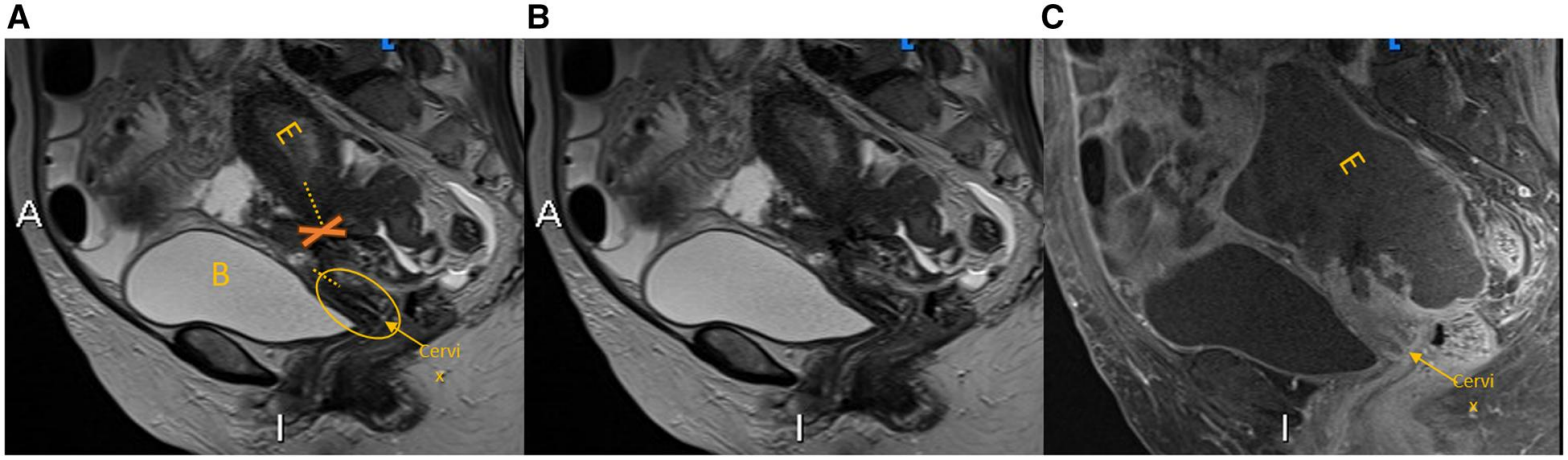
Day 12 of admission MRI pelvis with contrast—sagittal slices. (A and B) *T2-weighted sagittal sequence, both annotated and unannotated*. A (annotated) demonstrates the high T2 signal bladder anteriorly, with the Uterus and T2 bright Endometrial cavity (E) posterior and superior to the bladder. The “X sign” is again appreciable with uterine and adnexal structures tethering between the misaligned uterus and cervix. On A, the cervix and uterine body/fundus (E), do not appear to normally align, with discontinuation of the alignment represented by the dotted lines. (C) *Post-contrast sagittal T1-weighted fat suppressed image at the slice as A and B*: The cervix has again been labelled. The key finding on this image, is the total lack of normally expected uterine and uterine mass enhancement, whereas the misaligned cervix continues to demonstrate enhancement. Abbreviations: B = Bladder; Cervix = labelled; E = Endometrial cavity; X = “X sign”; Dotted line = alignment of uterus and cervix.
